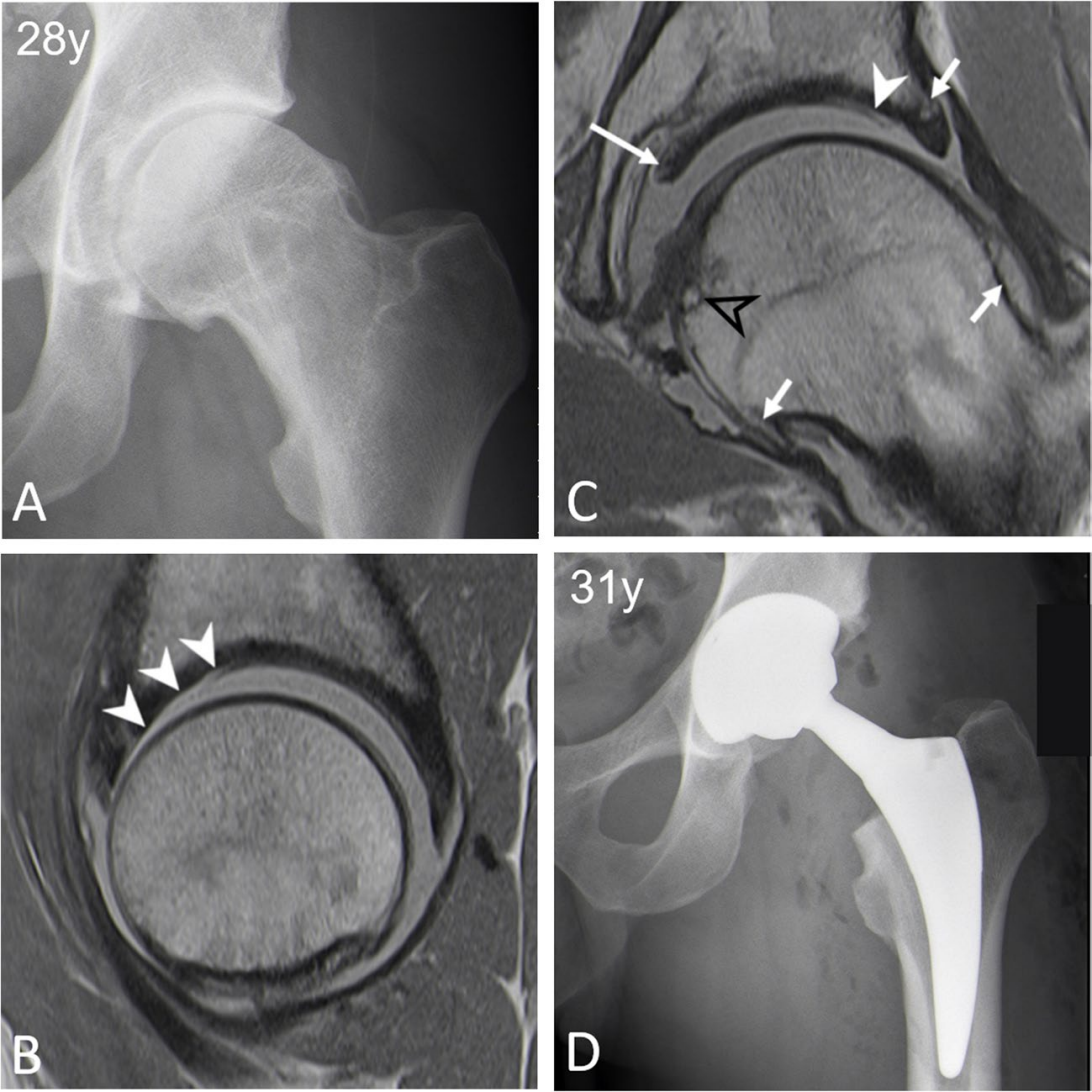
A 28-year-old man with cam FAI. B Preoperative traction MR-arthrogram with proton density-weighted turbo spin echo images showing two MRI findings independently associated with failure of FAI surgery. Sagittal- (B) and coronal (C) images show extensive cartilage defects involving the anterior- and superior quadrants (arrowheads). C The coronal image further demonstrates the central femoral osteophyte (black arrowhead), a central acetabular osteophyte (long arrow), as well as peripheral acetabular/ femoral osteophytes (short arrows). D Postoperative radiograph following total hip replacement 3 years after hip arthroscopy
